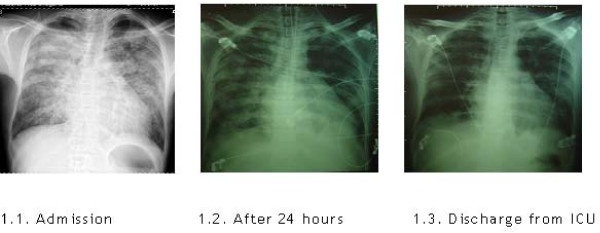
Chest X-ray evolution (admission to discharge from ICU). Increased heart-thorax index and heterogeneous diffuse infiltrate sparing upper lobes and bases. Favorable radiological evolution.
